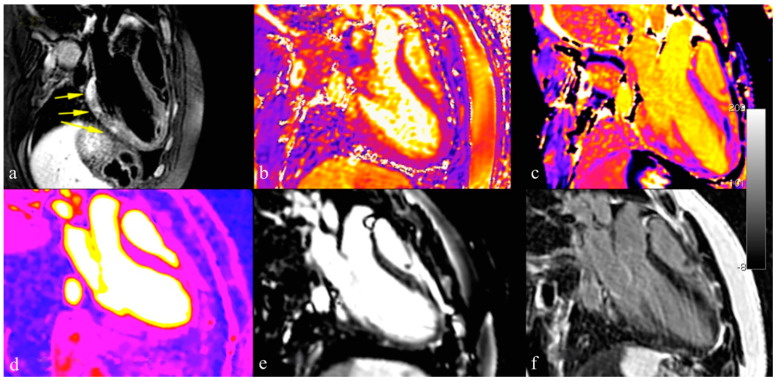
Different techniques for myocarditis assessment with cardiovascular magnetic resonance imaging: T2 STIR sequences reveal areas of myocardial edema in acute setting (panel a); T1 and T2 mapping techniques improved detection of myocardial edema and inflammation (panel b,c); perfusion imaging and early enhancement can be used to detect hyperemia condition (panel d,e); late gadolinium enhancement sequences confirmed acute myocardial inflammatory damage and assessment of myocardial fibrosis (panel f).
